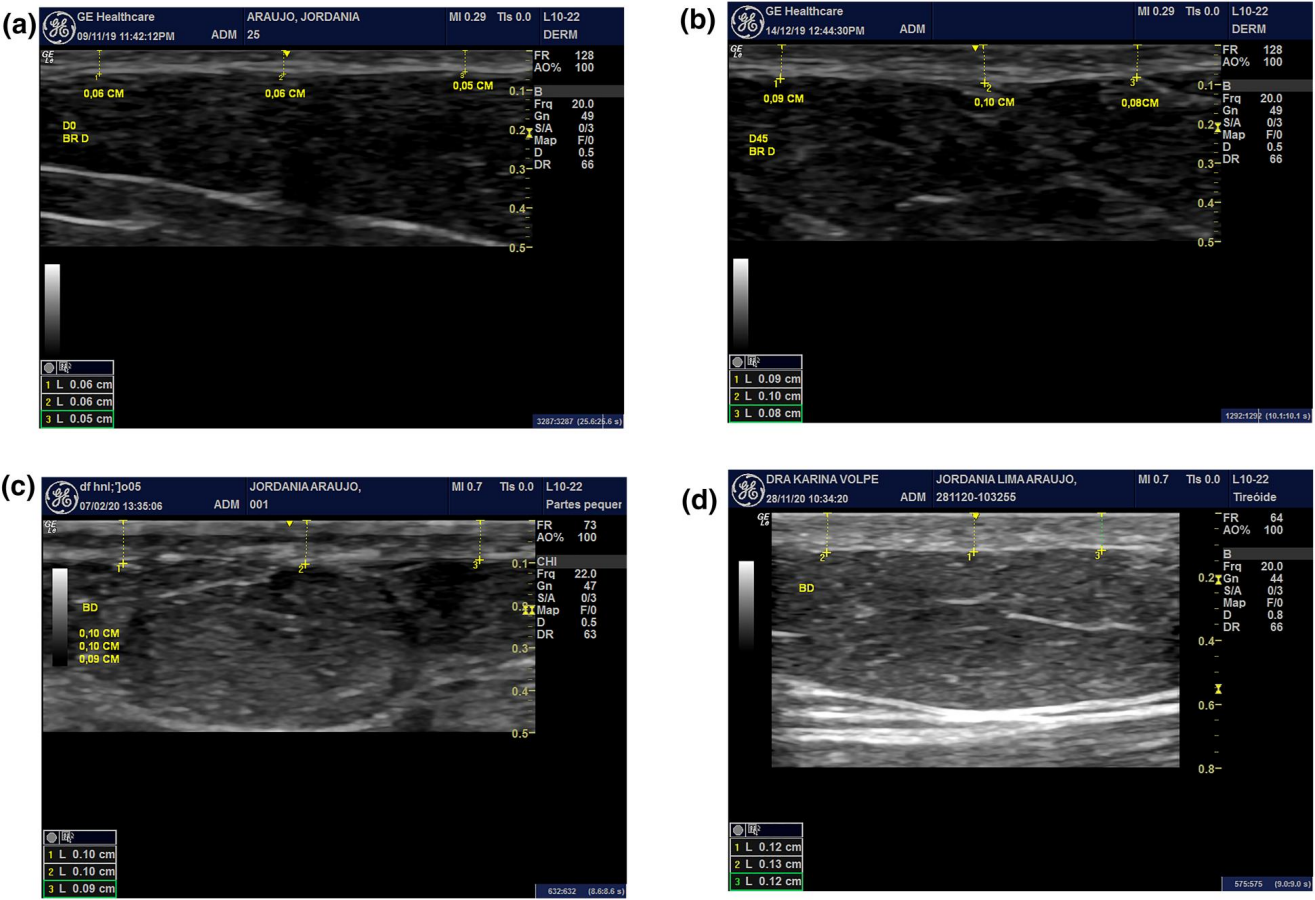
(a) Ultrasounds in the periods A—pre‐treatment (D0). (b) 45 days after the first application (D45). (c) After 45 days of the second application (D90). (d) 365 days after Do (D365)
